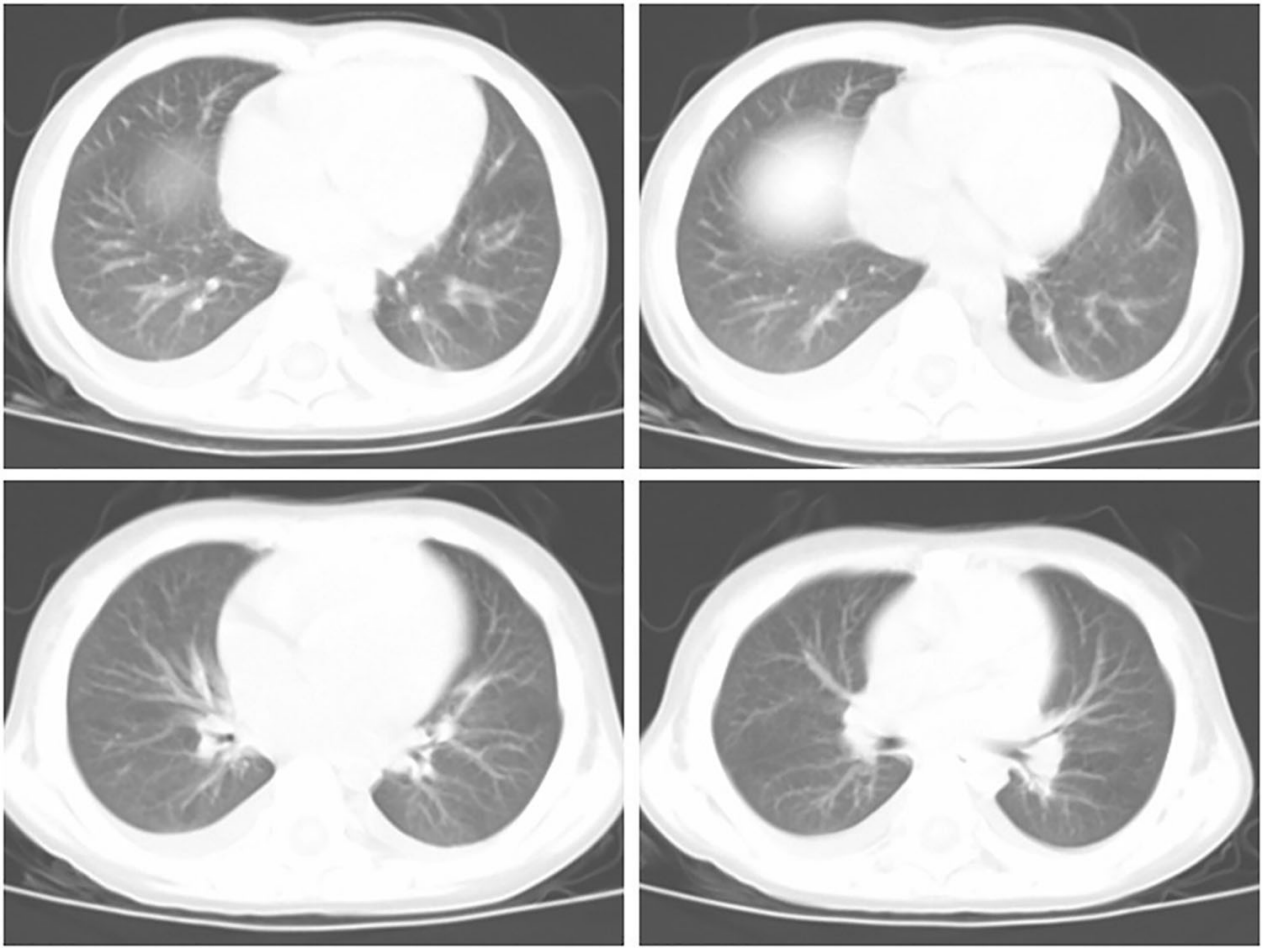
The review of chest CT after treatment in the pediatric patient. The bilateral lung lesions and pleural effusion were significantly absorbed and reduced, and the abdominal CT revealed no abnormalities. CT, computed tomography.
